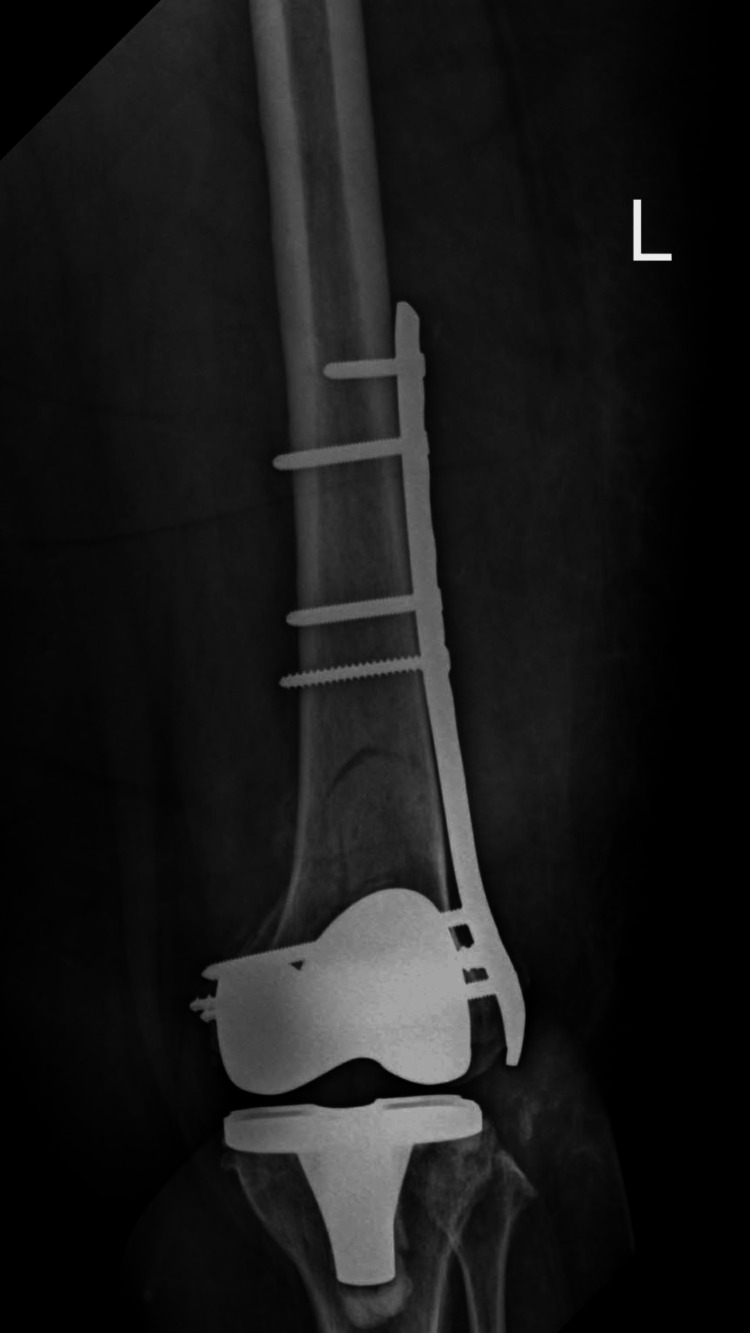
Postoperative radiograph demonstrating fixation of the periprosthetic distal femoral fracture with a distal femoral locking plate.
